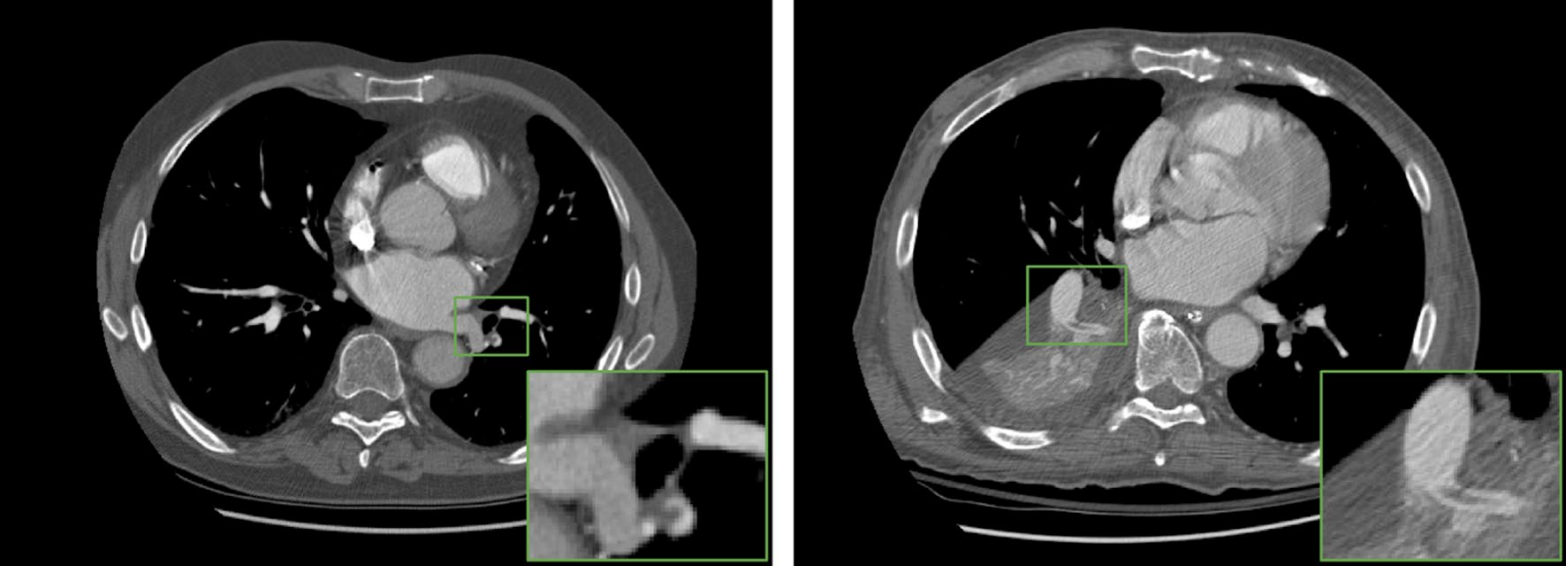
Two selected axial CT images of the chest from two separate patients with positive diagnosis of PE. The left CT scan demonstrates a left lower lobe posterolateral basal segmental artery filling defect consistent with a pulmonary embolism. The CT scan on the right panel demonstrates a small elongated filling defect bridging across the segmental arteries of the right lower lobe consistent with a segmental pulmonary embolism, in addition to surrounding collapse of the right lower lobe. The vision-only model yielded false-negative predictions for both cases, but the fusion model correctly predicted both as positive.
